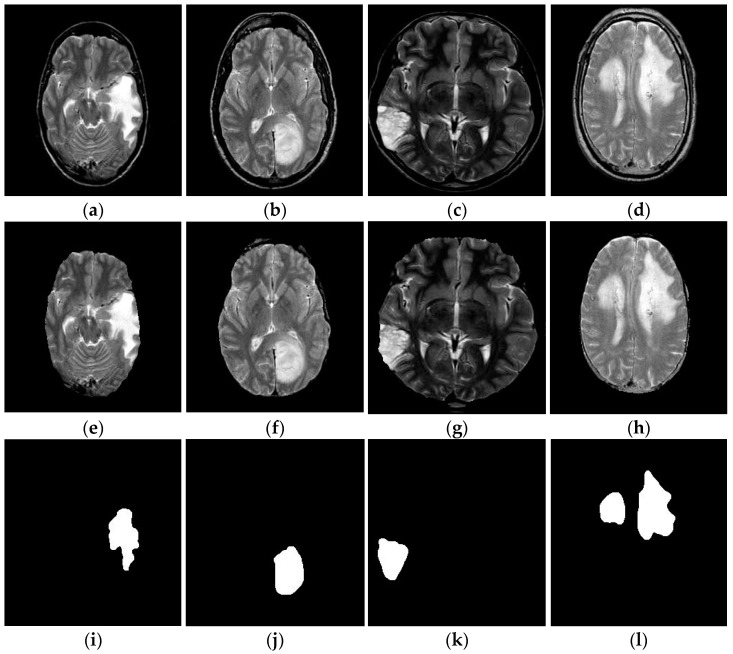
Outcomes of the proposed brain tumor segmentation: (a–d) Original brain tumor MR images. (e–h) Skull-stripped images. (i–l) Infected tumor region of the brain MR images by FL-MSCM.
